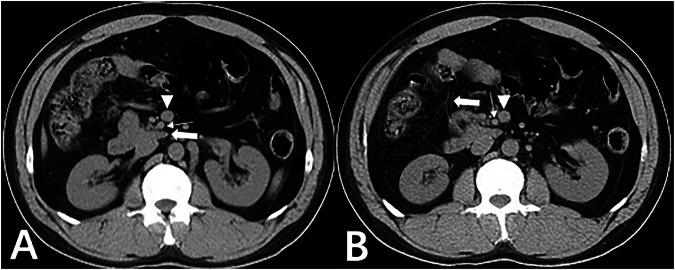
Twenty-two-year-old man with midgut malrotation. A Axial CT plain scan showed the horizontal segment of the duodenum (thick arrow) not traversing behind the SMA (thin arrow), instead curving rightwards and forwards adjacent to the SMA, forming a blind end; the SMV (solid triangle) located anterior to SMA. B Axial CT scan showed high position and anterior displacement of the cecum and appendix (thick arrow), with the SMV (triangle) rotating clockwise around the SMA (thin arrow)
